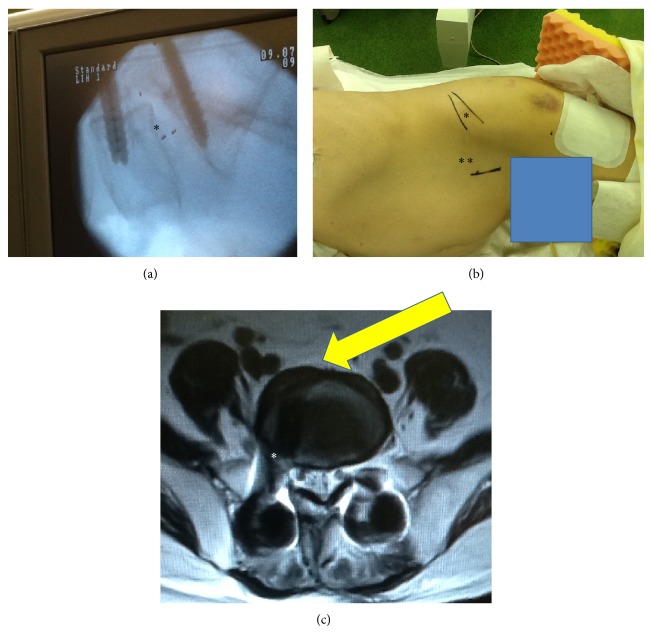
(a) Posterior movement of the TLIF cage 6 months after surgery (^*^). (b) The patient was placed in a lateral decubitus position. ^*^Midportion of the L5-S1 disk. ^^A 5 cm skin incision was made 10 cm anterior from the midportion of the L5-S1 disk. (c) MRI showing right foraminal stenosis between L5 and S1 because of the cage. Arrow indicates the approach between major vessels.
